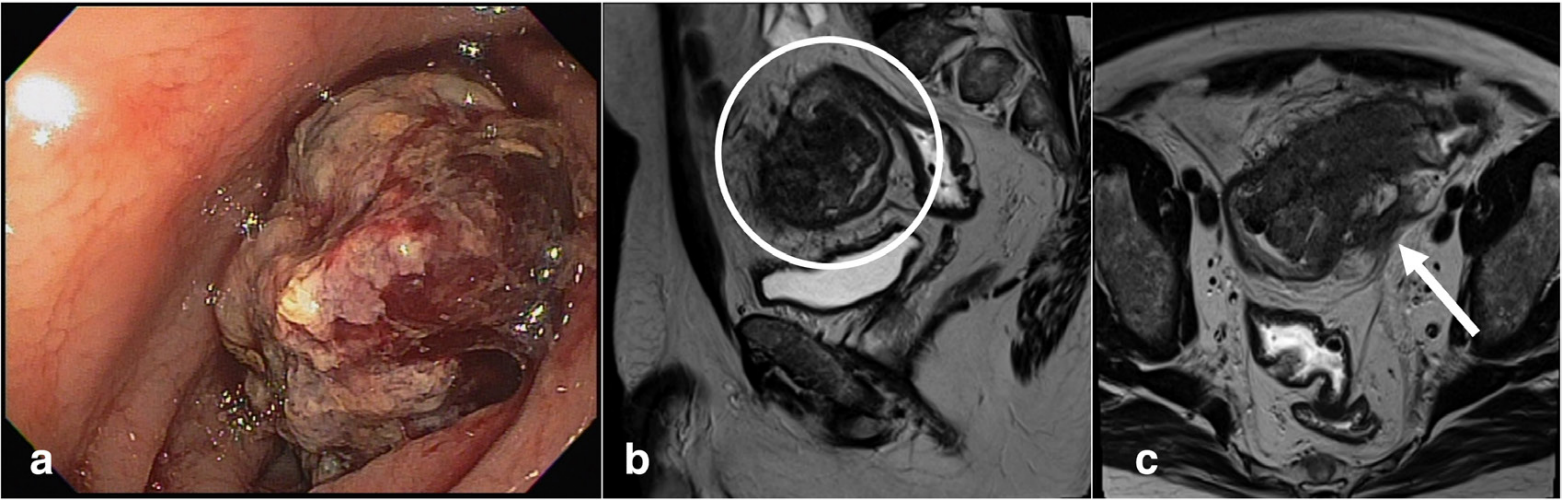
Endoscopic assessment showing vegetative and friable lesion in the distal sigmoid colon (a). Baseline staging MRI showing infiltrative and stenosing lesion of the middle / distal third of the sigmoid in sagittal (b) and axial (c) planes with infiltration of mesosigmoid fat up to peritoneal reflection (arrow).
